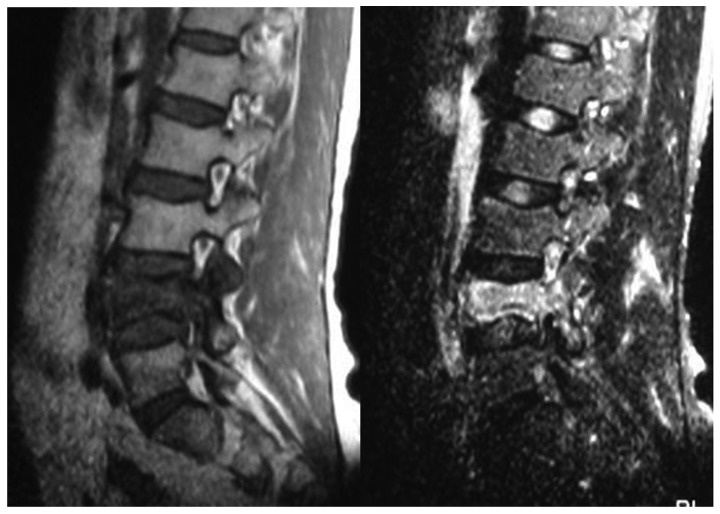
MRI, sagittal section. Hypointensity on T1-W images of L4 planar appearance with a mild compression fracture. T2-W images reveal more marked hypersignals of the body of L4 associated with the recent collapse. MRI, magnetic resonance imaging; L4, fourth lower lumbar vertebra, T1 W, T1 weighted; T2 W, T2-weighted.
